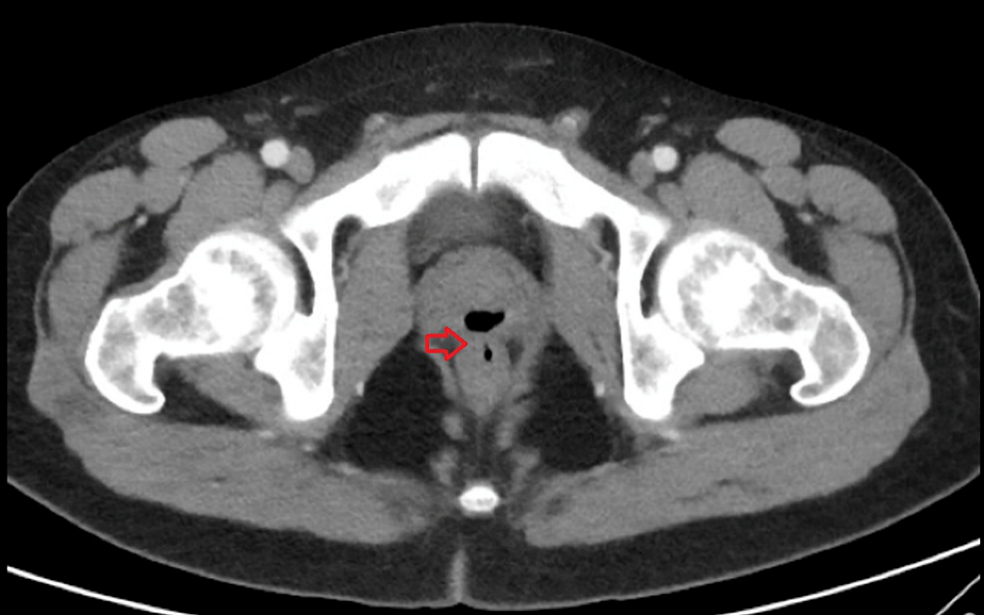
CT demonstrating gas-filled structure within the posterior aspect of the prostate communicating with the adjacent rectum. CT: computed tomography
